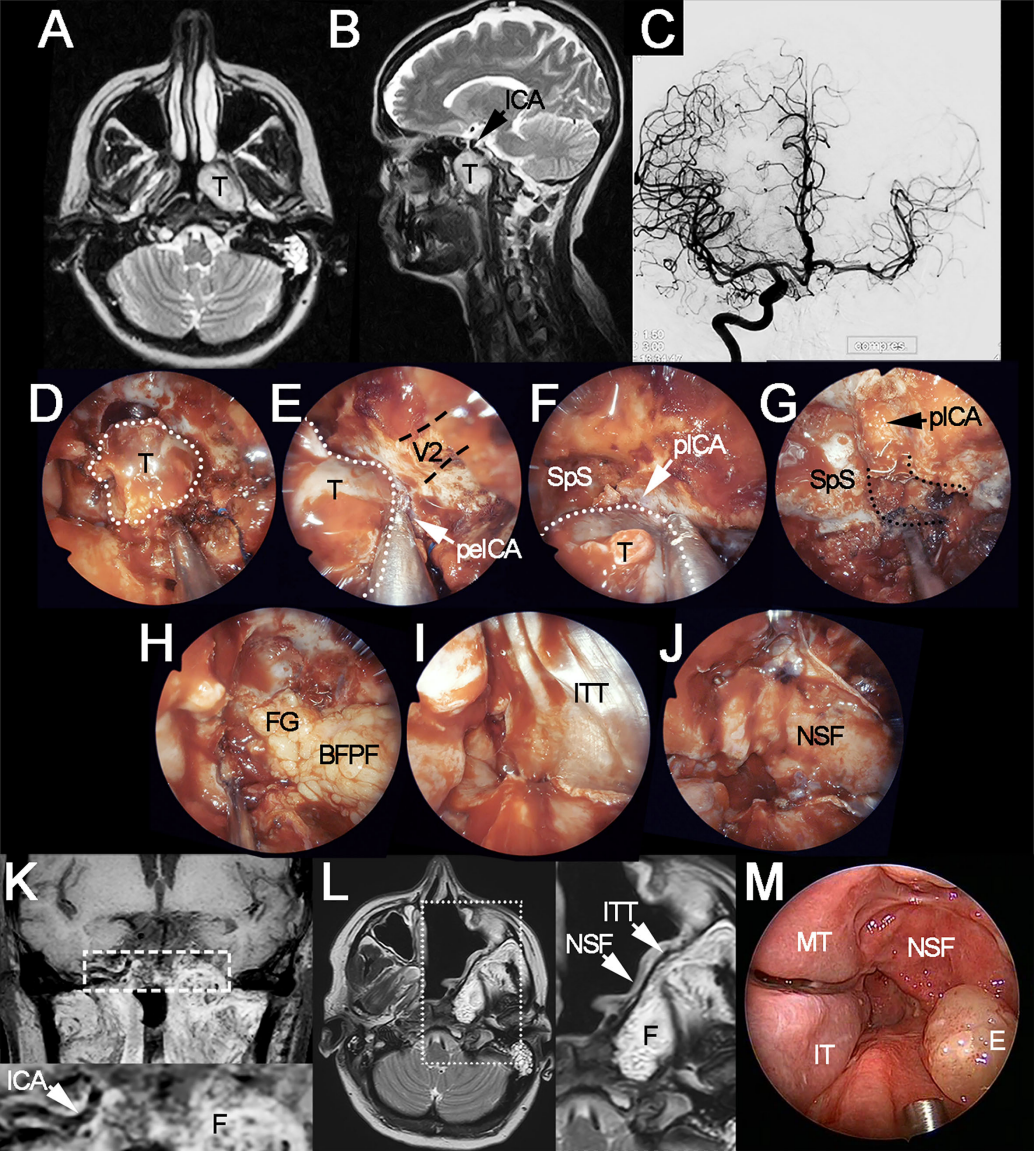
Locally advanced polymorphous adenocarcinoma of the left nasopharyngeal wall-sphenoid sinus, treated through left extracranial-to-intracranial bypass surgery, endoscopic transnasal resection, and adjuvant intensity-modulated radiation therapy. (A, B) Preoperative axial and sagittal T2-weighted MRI showing the tumor (T) and its spatial relationship with the internal carotid artery (ICA). (C) Angiography of the temporary balloon occlusion test, which showed adequate crossflow timing but was considered as positive for ischemia due to neurological signs at sensitization through drug-induced hypotension. (D) Endoscopic appearance of the tumor (white dotted line) after sphenoidotomy, ethmoidectomy, and medial maxillectomy. (E, F) Intraoperative evaluation confirmed the presence of tight adhesion to the caudal paraclival (pICA) and medial petrous (peICA) tracts of the internal carotid artery. (G) Endoscopic view of the surgical field following carotidectomy, occlusion coils can be sees as emerging from the distal stump of the vessel (i.e., cranial paraclival tract). Clearance of the carotid canal (black dotted lines) and petroclival junction have been performed. (H–J) Reconstruction of the skull base defect through the right buccal fat pad flap (BFPF), fat graft (FG), iliotibial tract graft (ITT), and the right nasoseptal flap (NSF). (K) Coronal T1-weighted, fat-saturated, contrast-enhanced MRI acquired 10 months after surgery (7 months after completion of adjuvant radiation therapy). White dashed rectangle indicates the position of magnification at the bottom of the image, which shows the right internal carotid artery and enhancing fat (F) in the position of the left carotid canal and petroclival junction. (L) T2-weighted MRI acquired 10 months after surgery. White dotted rectangle indicates the position of magnification on the right of the image, which shows the layers of the reconstruction. (M) Endoscopic appearance of the surgical site 10 months after surgery. V2, position of the maxillary nerve (black dashed lines indicate the trajectory of the nerve); E, mucosal edema; IT, inferior turbinate; MT, middle turbinate; SpS, sphenoid sinus.
